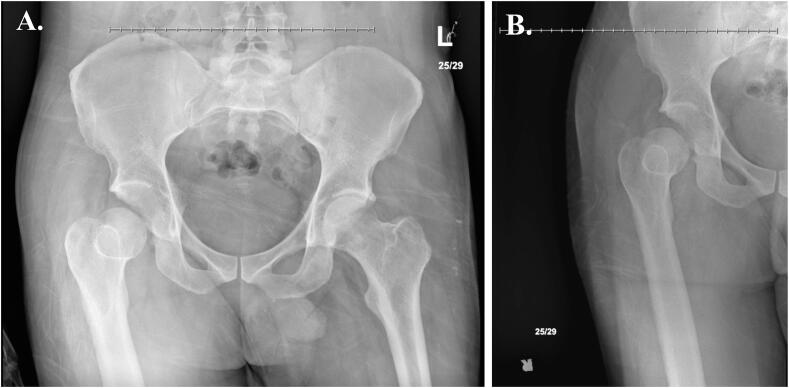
AP radiographic views of the (A) bilateral and (B) right hip demonstrating right hip posterior dislocation at initial presentation.
